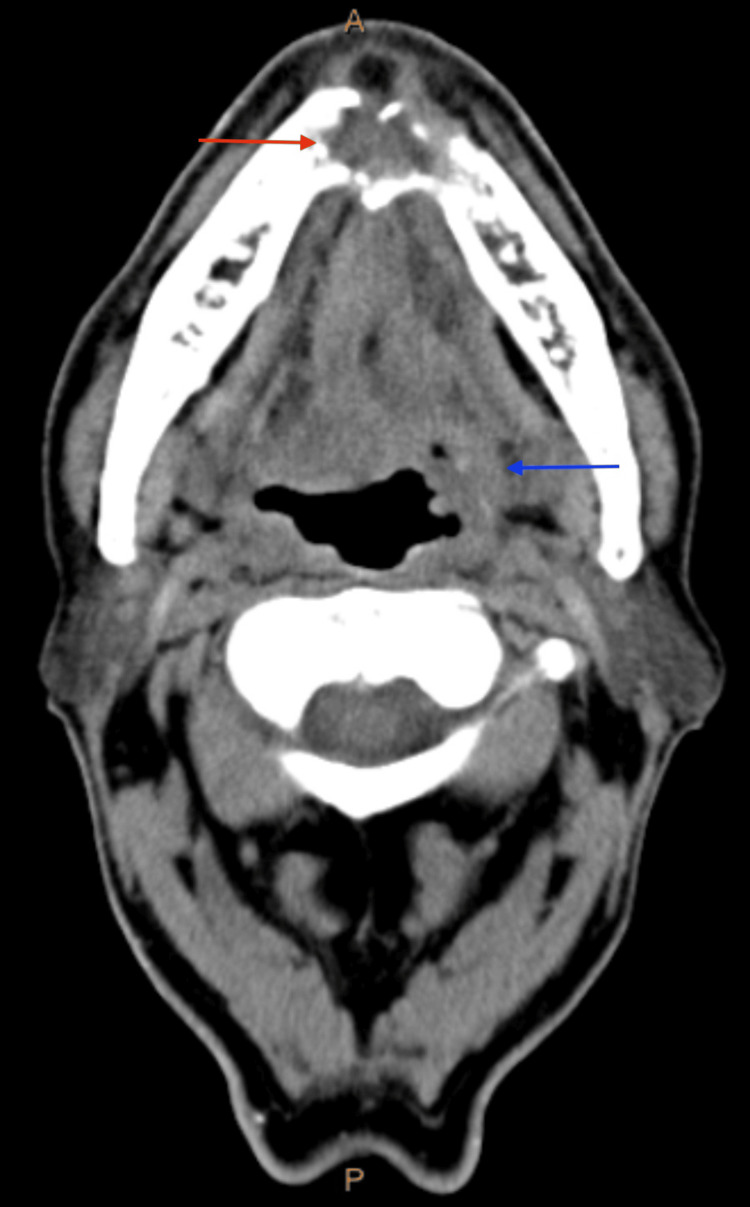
Post-induction Chemotherapy Axial CT Neck of Mandibular and Base of Tongue Mass Post induction axial CT neck with contrast showing worsening of soft tissue mass in the mandibular symphysis (red arrow) and decreased size of the left base of tongue mass (blue arrow)
